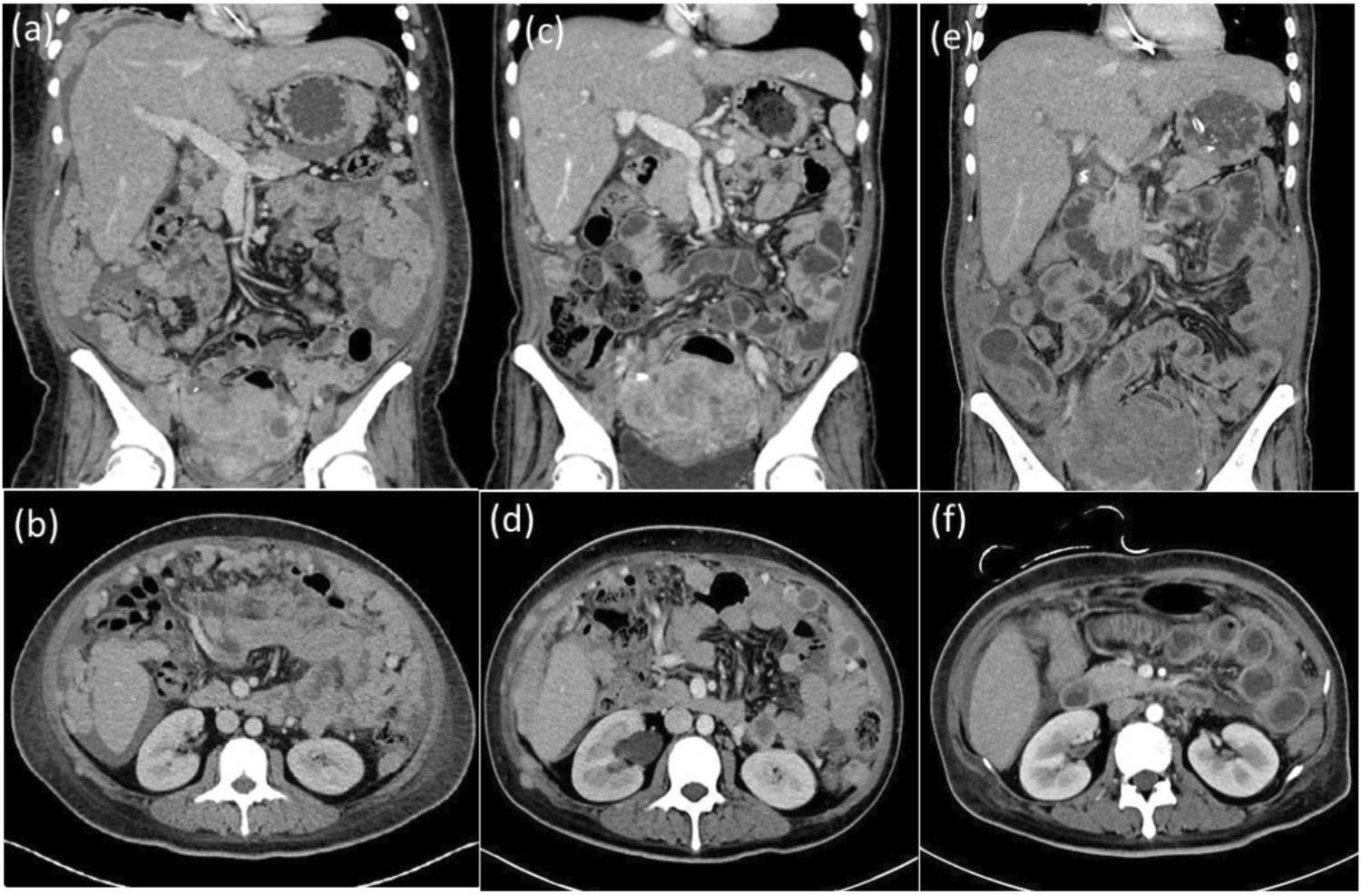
Coronal view a and c and transverse view b and d of abdominal and pelvic computed tomography. a and b Before treatment: bilateral adnexal masses with diffuse lymphadenopathies in the mesentery, multiple peritoneal tumors over the omentum, and peritoneum. c and d After treatment: tumor shrinkage and partial regression of plasmablastic lymphoma after chemotherapy plus target therapy; e and f showed stationary lymphadenopathies with interval regression after halpo-PBSCT
